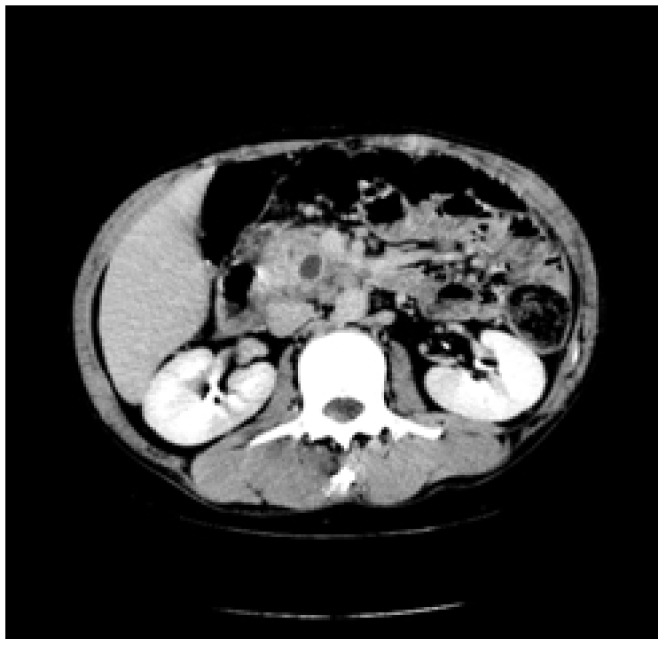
Enhanced CT revealing enlargement and cystic low-density shadow of head of pancreas.
